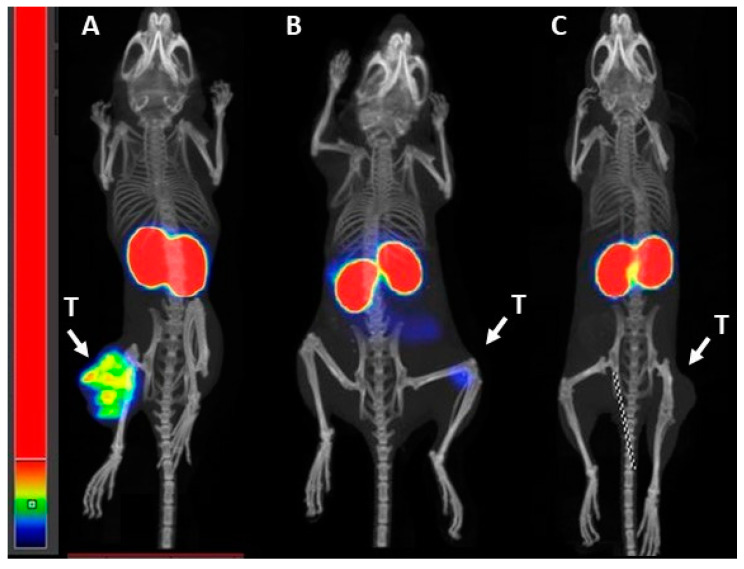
Representative nanoScan SPECT/CT images of PC3-pip xenograft (A) compared with PSMA-negative PC-3 (B) and SKOV-3 (C) xenografts after injection with [^99m^Tc]Tc-BQ0413 (40 pmol, 1 MBq) at 3 h pi. Arrows point at tumors (T).
